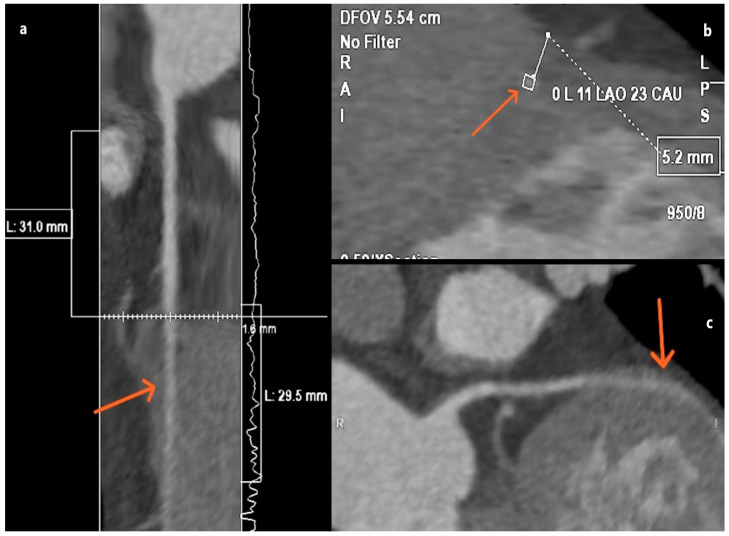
MD CT angiography plane images (a–c) showing a 5.2 mm (measurement in (b)) coronary myocardial bridge on LAD (arrow in (a–c)). Noted patient with a very deep myocardial bridge experienced pronounced anginal symptoms even with minimal exertion. The exercise stress echocardiogram was terminated within the first few minutes due to incidental ischemic findings. Management was predominantly conservative, consisting of lipid-lowering therapy with statins, along with beta-blockers and calcium channel blockers to reduce myocardial oxygen demand and alleviate symptoms. Due to the patient’s youth (early forties), invasive treatment (coronary artery bypass grafting CABG) was advised only if angina symptoms were to continue in non-exertional circumstances.
